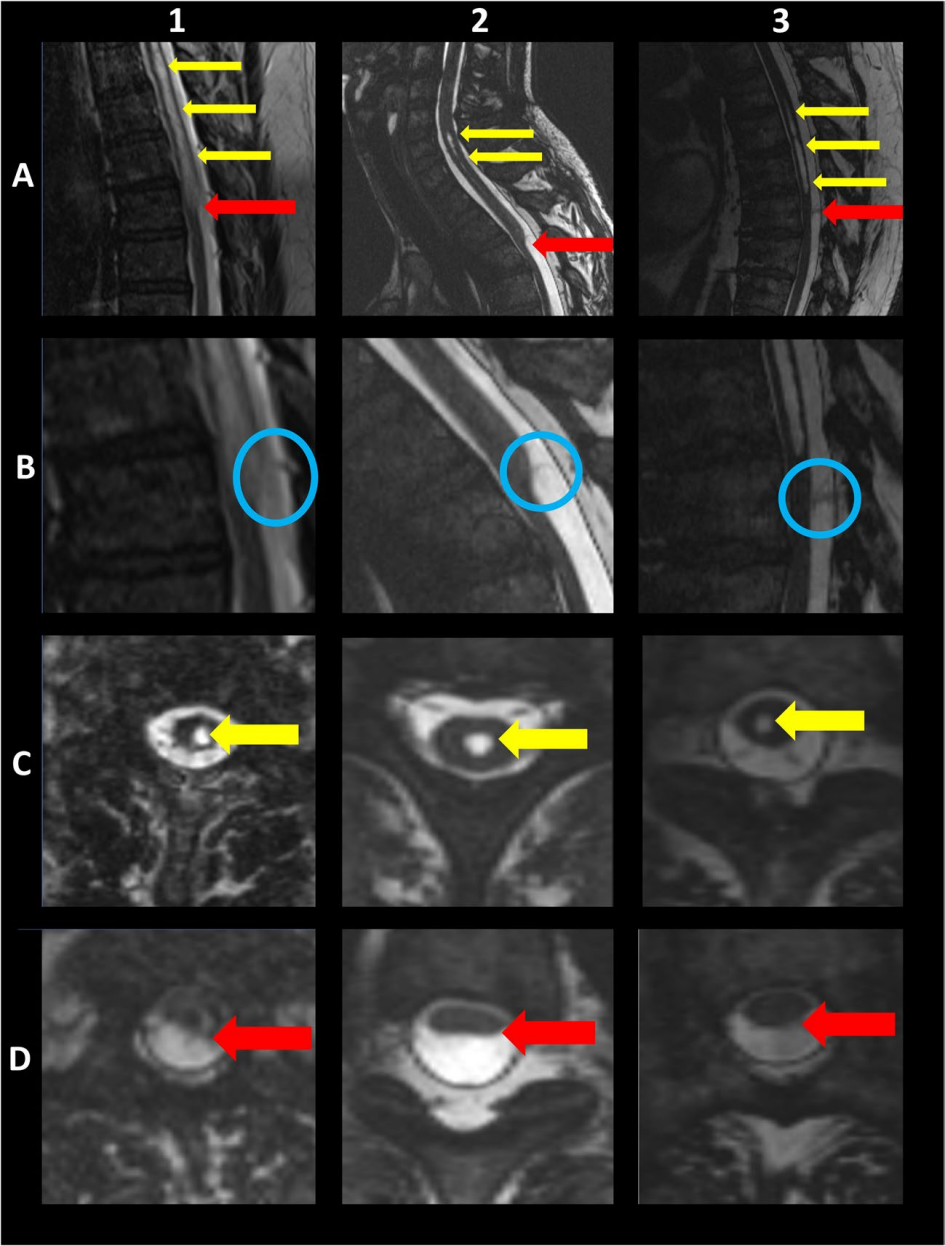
Preoperative magnetic resonance images of patient 1–3. A Sagittal MRI revealed displacement and caliber variation of spinal cord (red block arrows) and syringymyelia rostral to the SAW (yellow block arrows). B Sagittal MRI TrueFISP revealed a web in all three cases (blue circle). C Axial MRI TrueFISP of the syrinx (yellow block arrows). D Axial MRI TrueFISP of discplacemnt and caliber variation of spinal cord (red block arrows)
